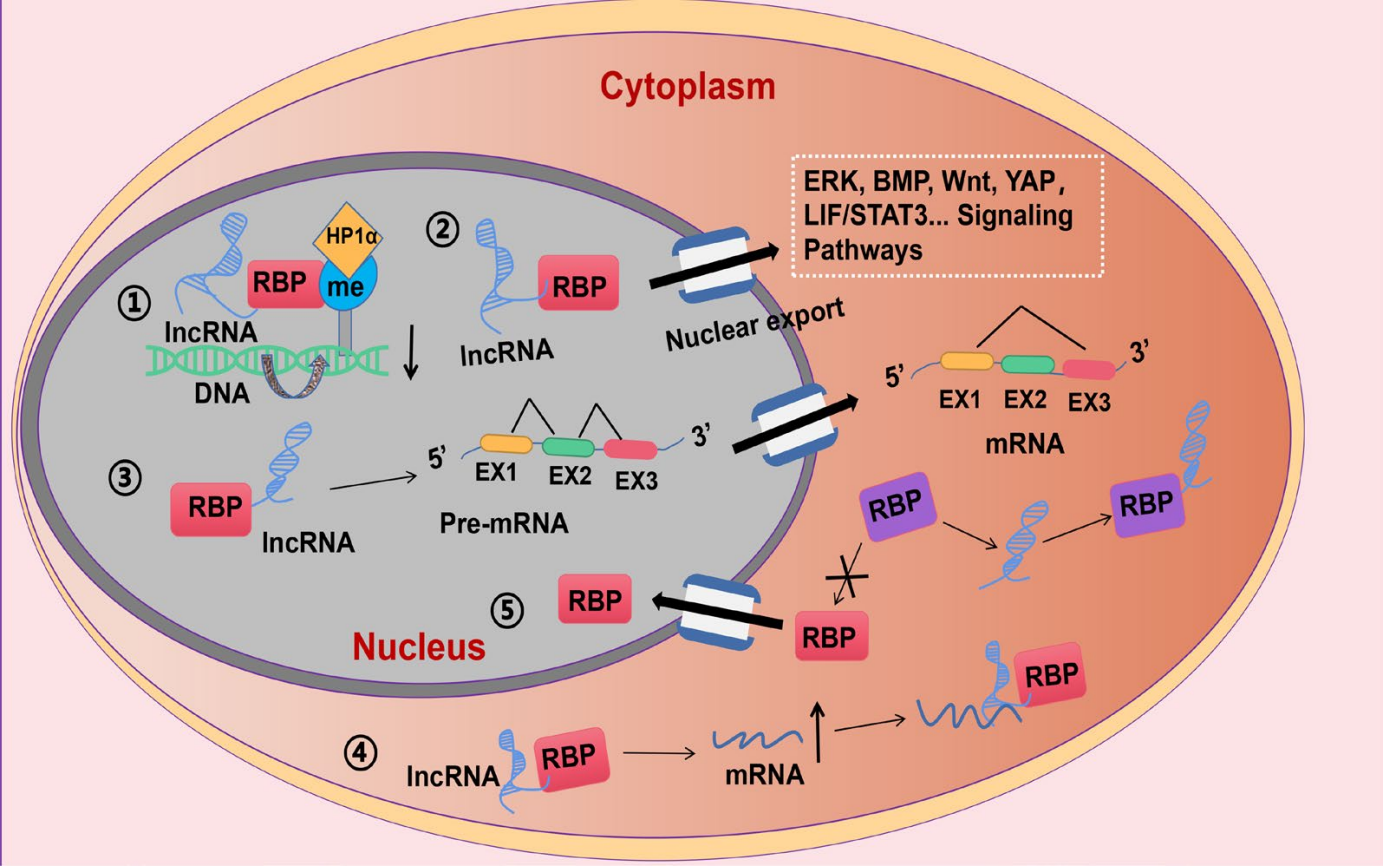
Functions of lncRNAs and their RBPs in self-renewal and differentiation of stem cells. (1) LncRNAs and their RBPs affect epigenetic regulation in stem cells. For example, up-regulated Oct4P4 combines with SUV39H1 HMTase to form a complex, which recruits H3K9me3 and HP1α to the Oct4 promoter region, resulting in Oct4 silencing and preventing mESC self-renewal. (2) LncRNAs and their RBPs are involved in the regulation of signaling pathways in stem cells. For example, lncR492 interacts with the mRNA-binding protein HuR and inhibits the neuroectodermal differentiation of mESCs by activating Wnt signaling. (3) LncRNAs and their RBPs regulate the splicing of mRNA in stem cells. For example, MALAT1 can be absorbed by hippocampal HT22 cells and promotes the splicing of PKCδII by binding the splicing factor SRSF2, which leads to the proliferation of neurons in the brain injury site of hASCs. (4) LncRNAs and their RBPs are involved in the regulation of the stability of mRNA in stem cells. For example, lncRNA ANCR binds to PTBP1, promotes the interaction between PTBP1 and inhibitor of ID2 mRNA and enhances the stability of ID2 mRNA, resulting in inhibition of the differentiation of hAMSCs into DE cells. (5) LncRNAs and their RBPs are involved in the regulation of the subcellular localization in stem cells. For example, lncRNA P53RRA inhibits the binding of G3BP1 and p53 in cytoplasm through specifically binding with G3BP1, so p53 is transferred from cytoplasm to nucleus to function as a transcription factor
